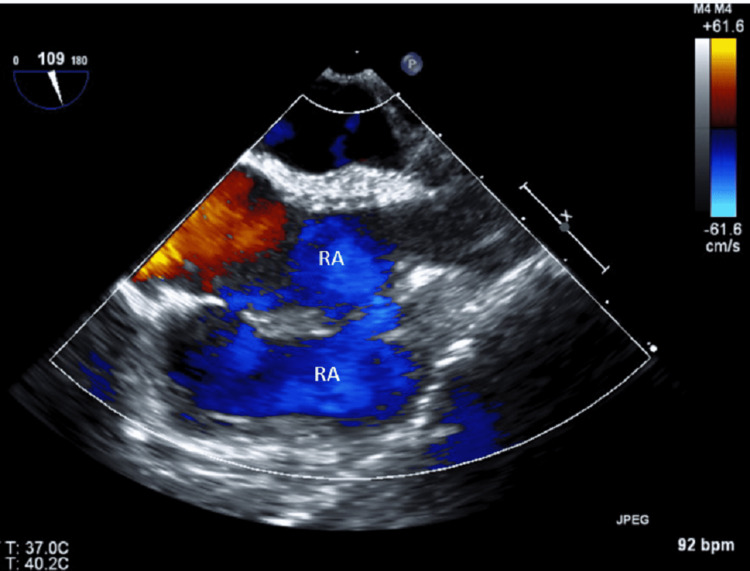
Trans-esophageal echocardiogram (TEE) Doppler film indicating the right atrium as a single cavity on both sides. RA, right atrium
